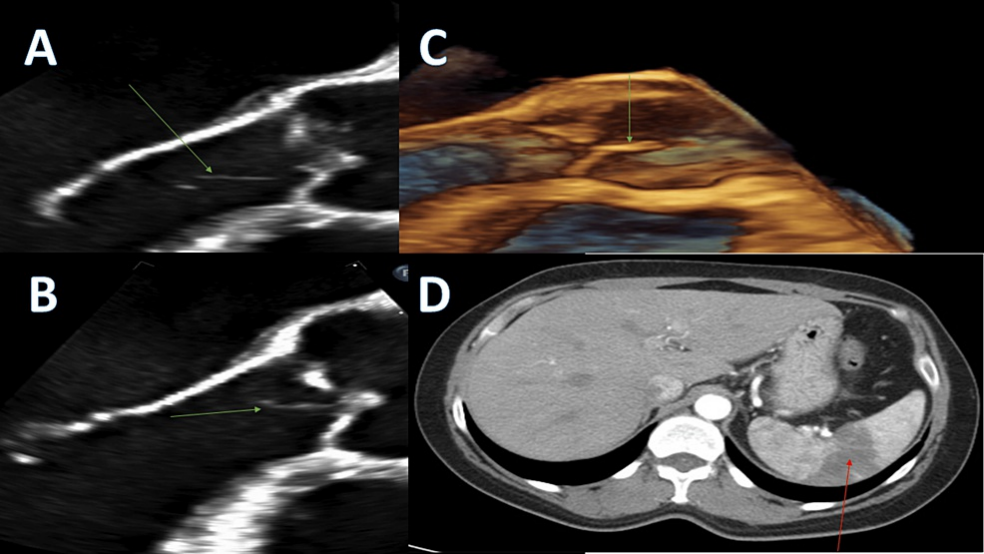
Images of vegetation and splenic infarct. A and B: Green arrow pointing to aortic vegetation on TEE, C: Green arrow pointing to aortic vegetation on TEE with reconstruction, D: Red arrow pointing to homogenous opacity on spleen indicating infarcted tissue TEE: transesophageal echocardiogram
